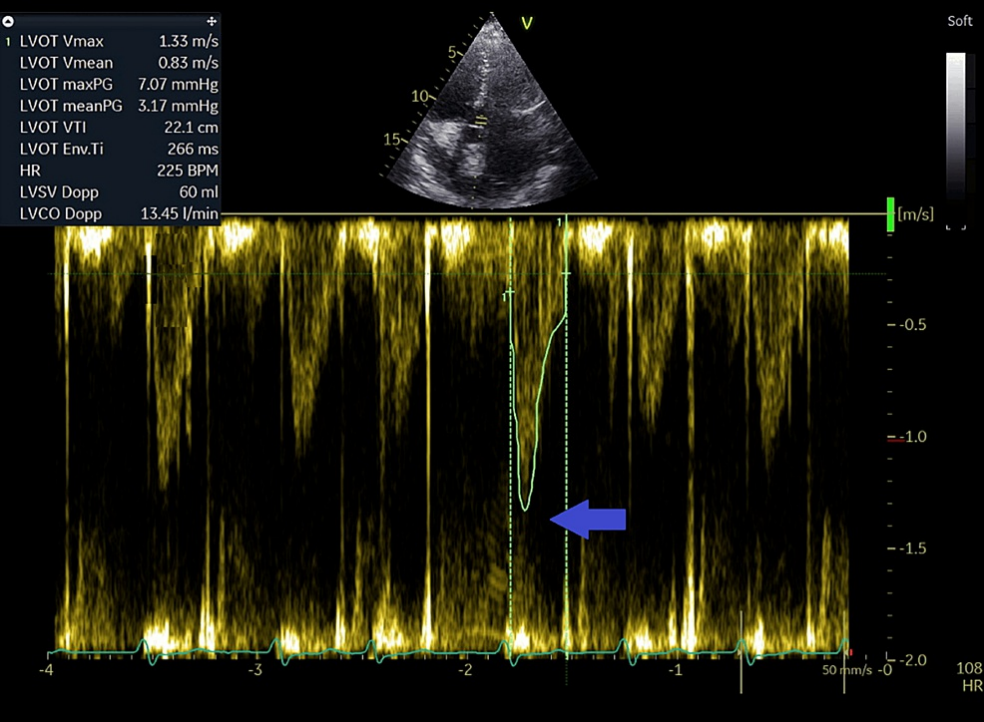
Measurement of the left ventricular outflow tract velocity at 1.3 m/seconds and time velocity integral at 21.1 cm from the apical five-chamber view (blue arrow).
